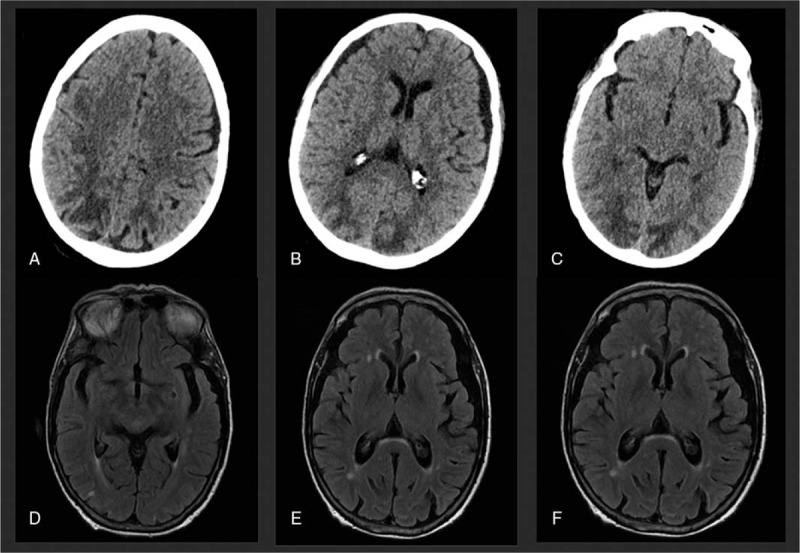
Case 2, CT showed hypodensities in the subcortical parieto-occipital white matter (A–C). Two weeks after treatment initiation, MRI FLAIR showed resolution of the lesions (D–F).
